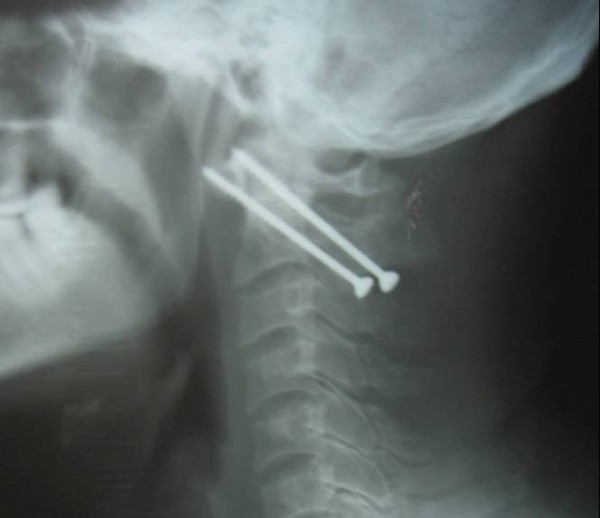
Postoperative radiograph of the subject showing placement of two transarticular screws across the reduced atlantoaxial joints.
